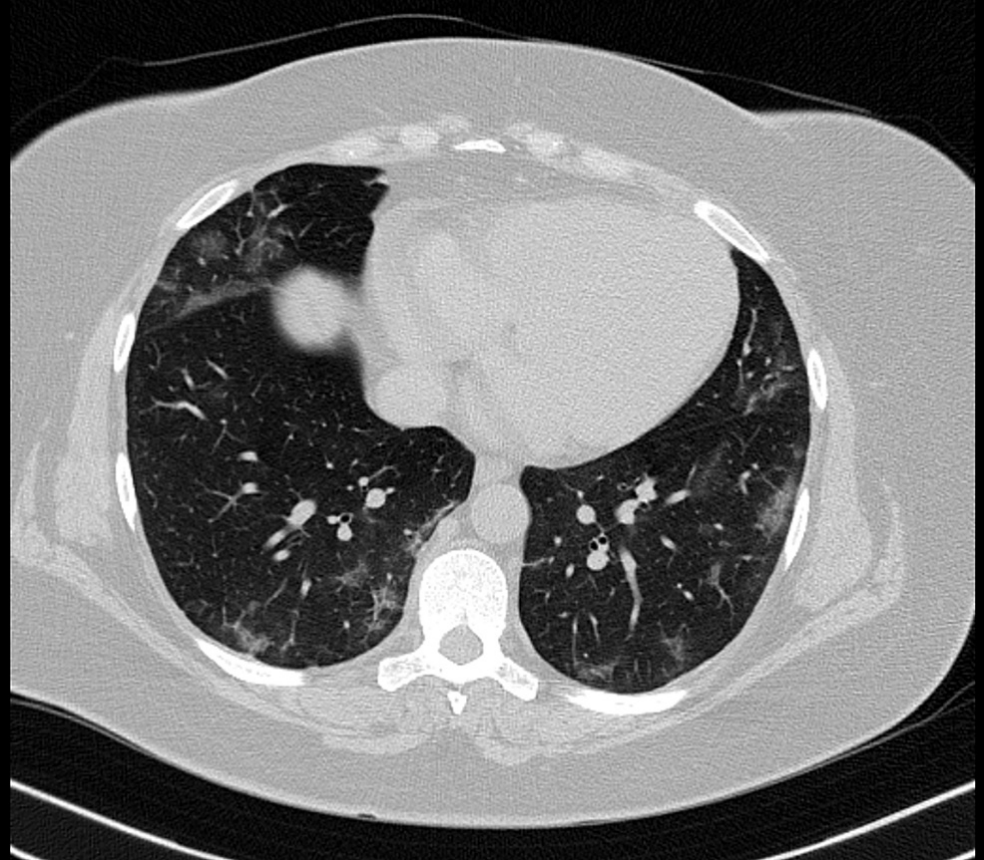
Non-contrast chest CT scan Multifocal, bilateral areas of ground-glass opacification are noted. Lesions were found predominantly in the inferior lobes. The percentage of lung involvement was approximately 5% by visual assessment.
